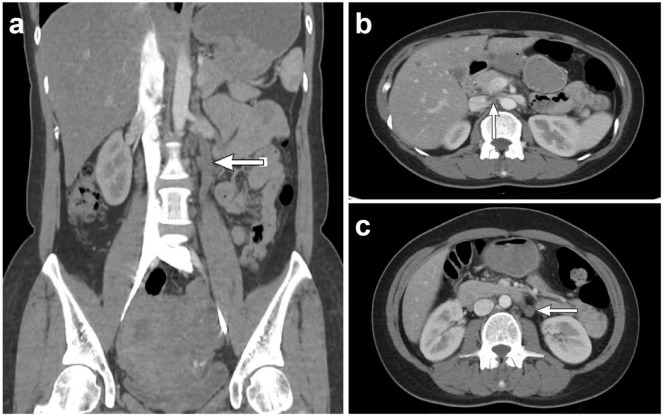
Computed tomography scan shows that when intravenous leiomyomatosis involves the parametrial/extrauterine blood vessels, thickening of the venous wall can be observed, accompanied by a cord-like filling defect [(a) left ovarian vein filling defect; (b) left renal vein and inferior vena cava filling defect; and (c) left ovarian vein filling defect].
